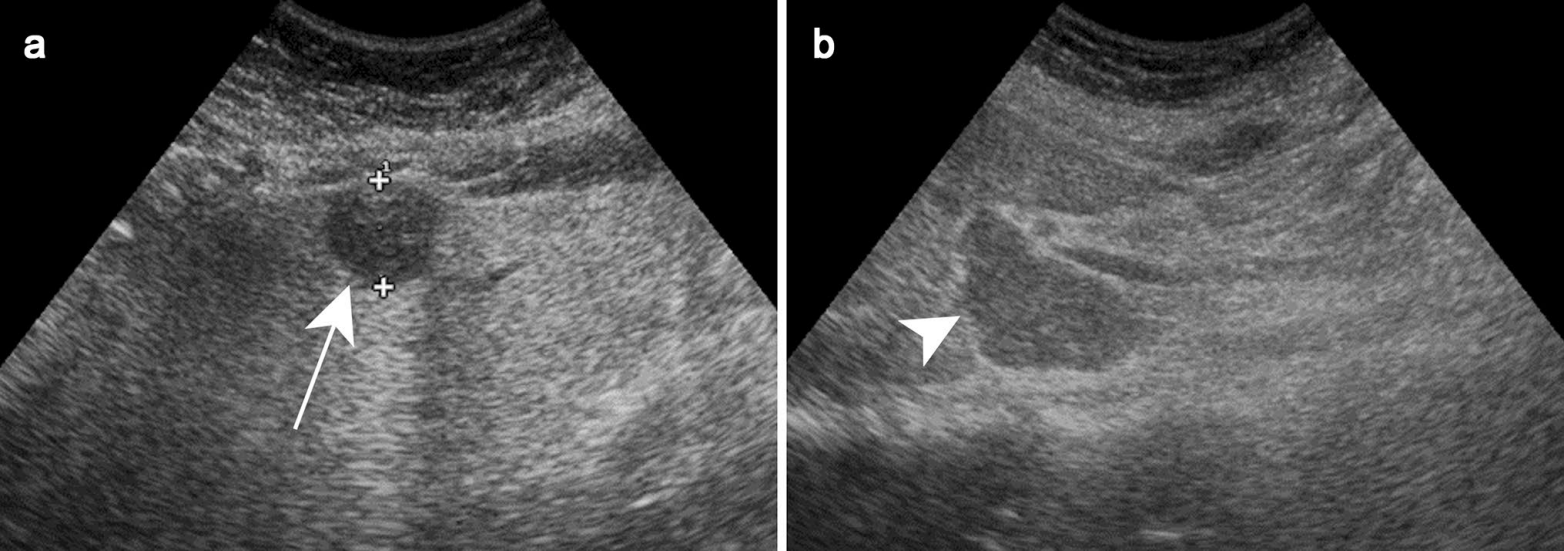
Liver tuberculosis in a 49-year-old female patient with asthenia and loss of weight without fever. Ultrasound a demonstrates a focal well-defined subcapsular hypoechoic lesion in segment III (white arrow). Enlarged lymph node (white arrowhead) is also observed in the porta hepatis (b) (Courtesy of Dr. Suzan Elhakiem, Ibn Sina Hospital, Khartoum, Sudan)
